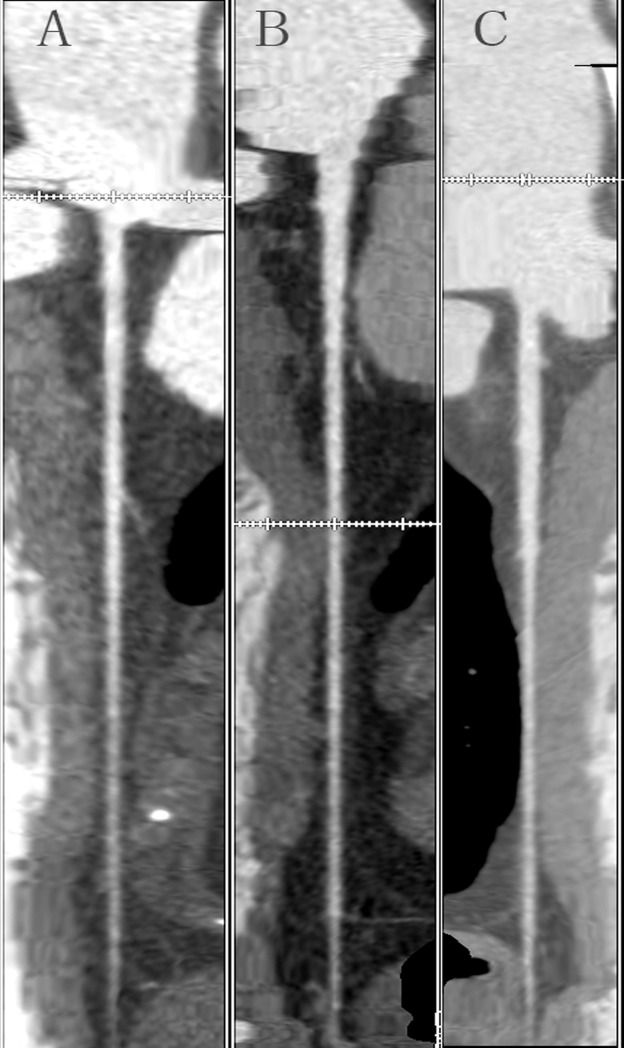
CTA of left anterior descending coronary artery in three patients using three protocols. A, 80 kVp, 300 mgI/ml; B, 100 kVp, 300 mgI/ml; C, 120 kVp, 370 mgI/ml with the same score: 4.30 rated by radiologists, which could be regarded as the same image quality.
